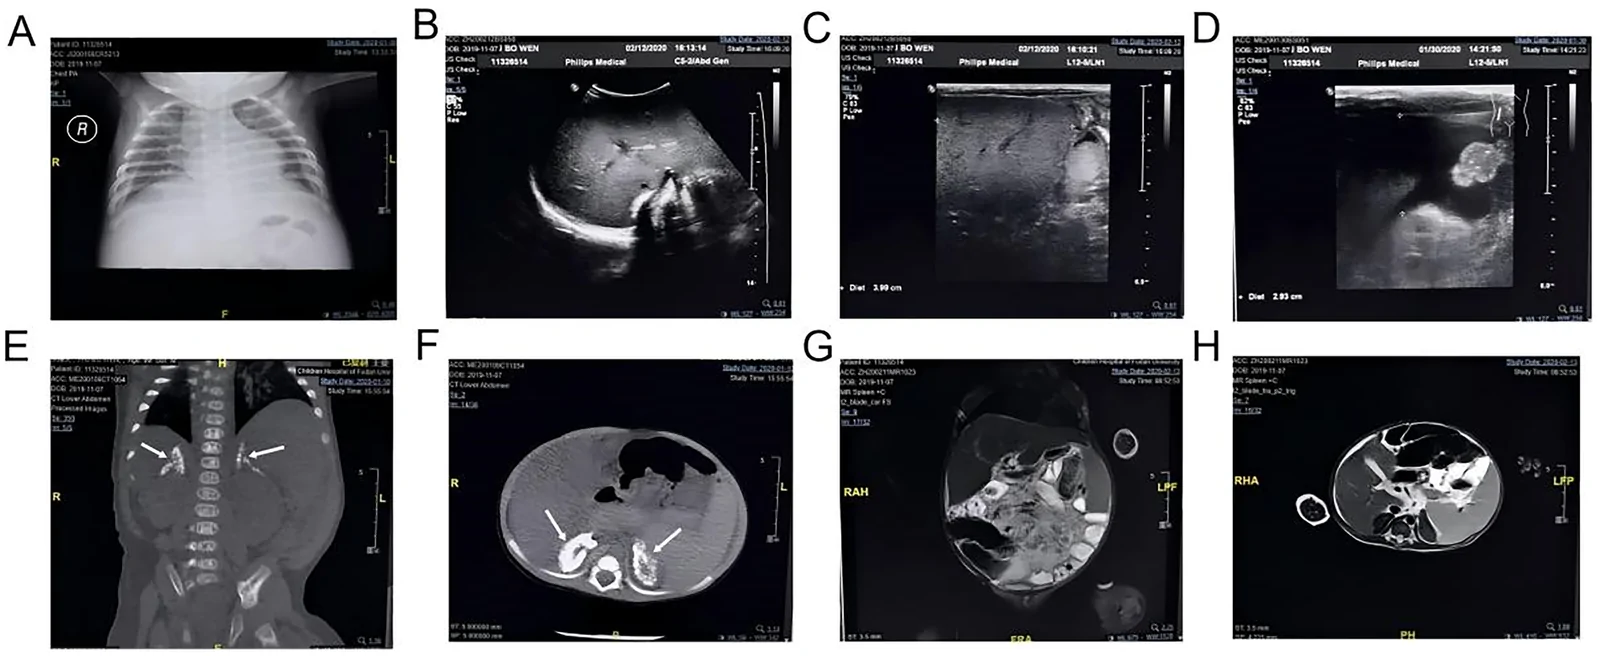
Imaging findings in Case 2. (A) Chest radiograph showing pulmonary congestion and pleural effusion. (B) Abdominal ultrasonography showing hepatomegaly. (C) Abdominal ultrasonography showing splenomegaly. (D) Abdominal ultrasonography showing ascites. (E) Abdominal computed tomography showing hepatosplenomegaly, abdominal distension, and bilateral adrenal calcifications (arrows). (F) Computed tomography demonstrating bilateral adrenal calcifications (arrows). (G) Abdominal magnetic resonance imaging showing marked abdominal distension and organomegaly. (H) Magnetic resonance imaging focused on the liver and spleen.
